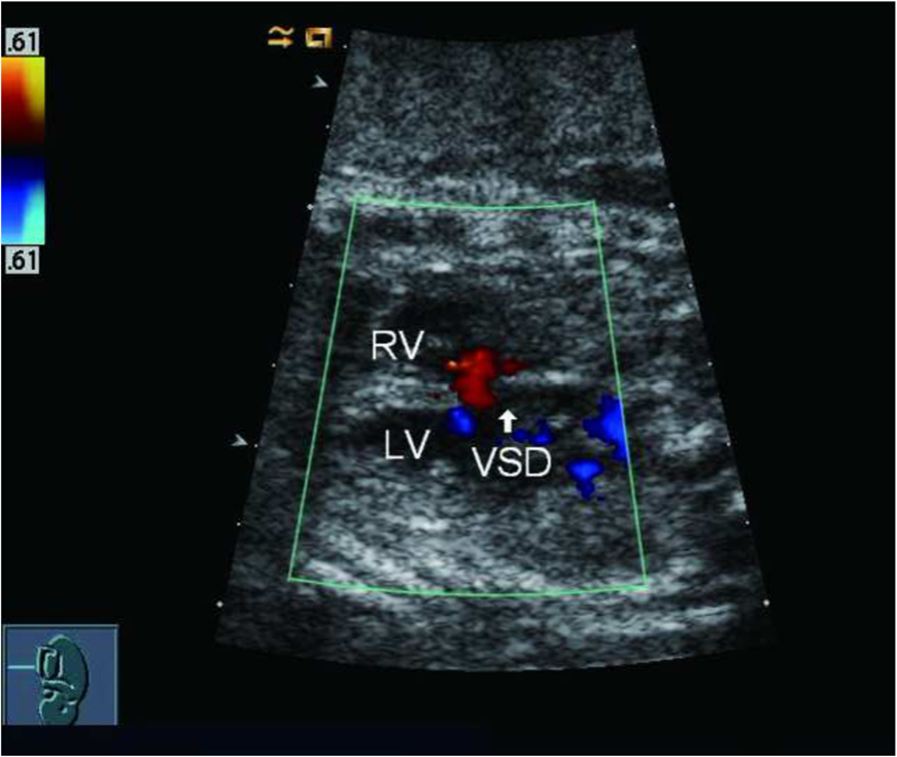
Color Doppler echocardiographic examination of large VSD
